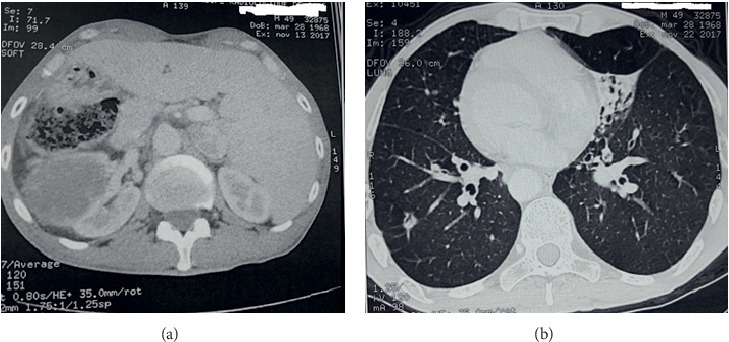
Computed tomography demonstrated a mass in the right kidney and mirror-image organs with left-to-right reversal (a) and bronchiectasia with situs inversus (b).
